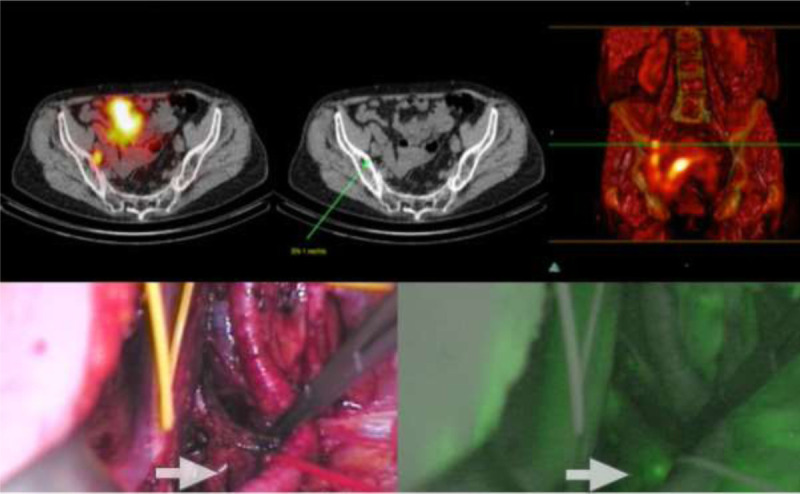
In the middle, transaxial fused SPECT/CT image of lymphatic drainage with SN seen above the normal ePLND area, in the aortic region. On the left, CT image of the same areas revealed a nonpathologic enlarged lymph node (arrow). On the right, volume-rendered fusion image of both scintigraphy SPECT and CT.
